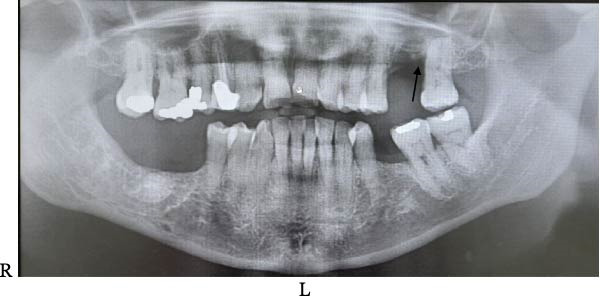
Panoramic radiograph taken 8 weeks postoperatively, showing an intact left maxillary sinus floor (arrow).
